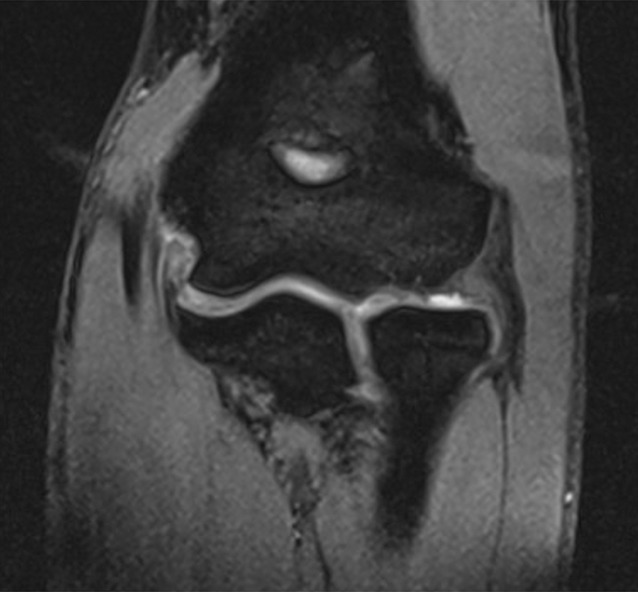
Magnetic resonance image of the elbow, showing a full-thickness tear of the medial collateral ligament. (Courtesy of the MoRe Foundation)
